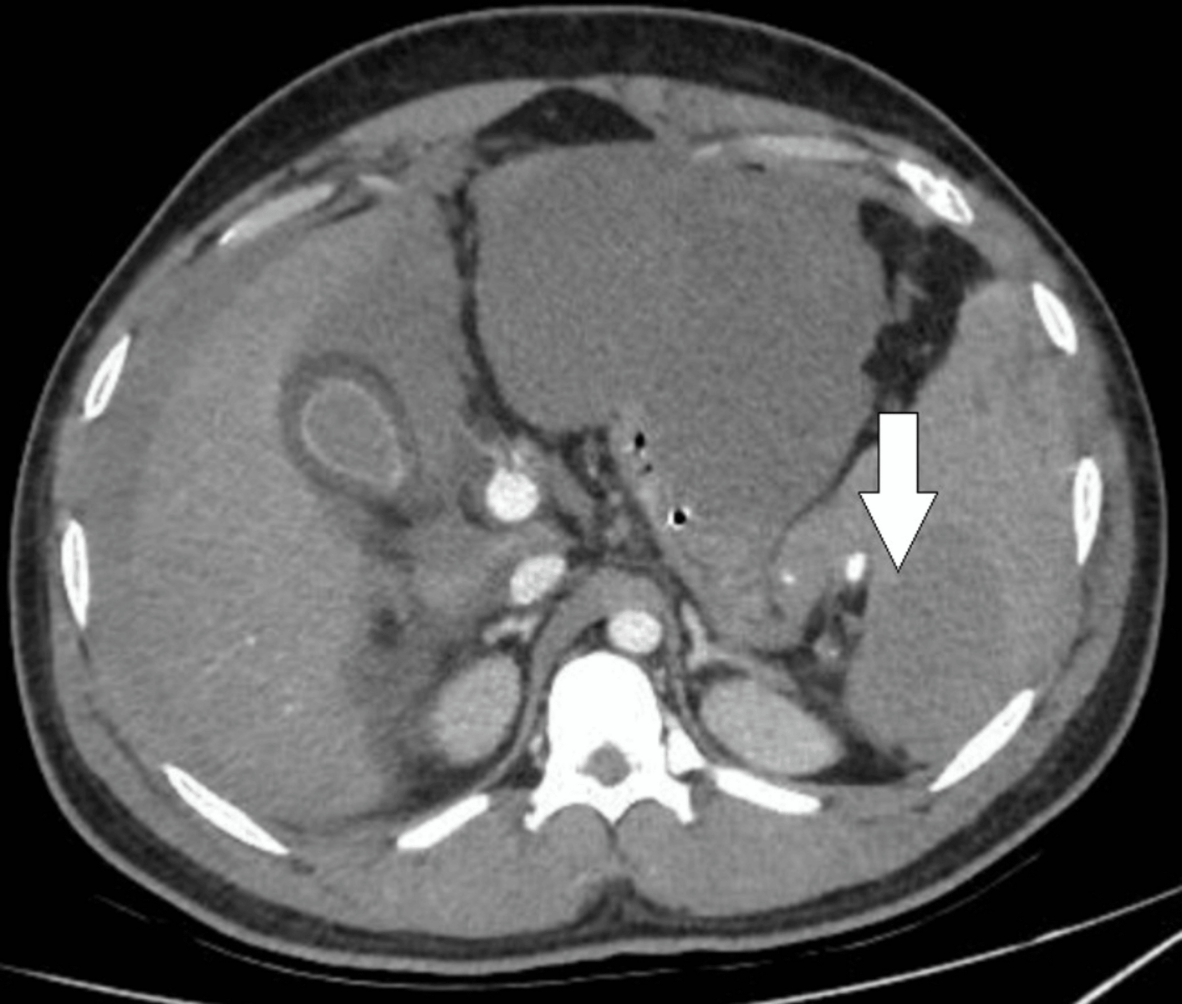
CECT abdomen on day 2 CECT: Contrast-enhanced computed tomography
